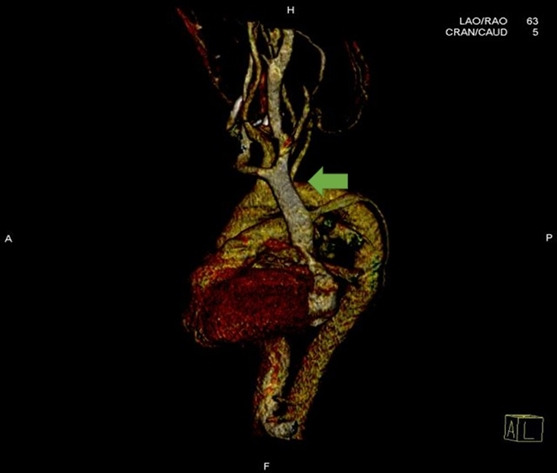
Computed tomography angiography, venous phase – three-dimensional view of persistent left superior vena cava (green arrow)
